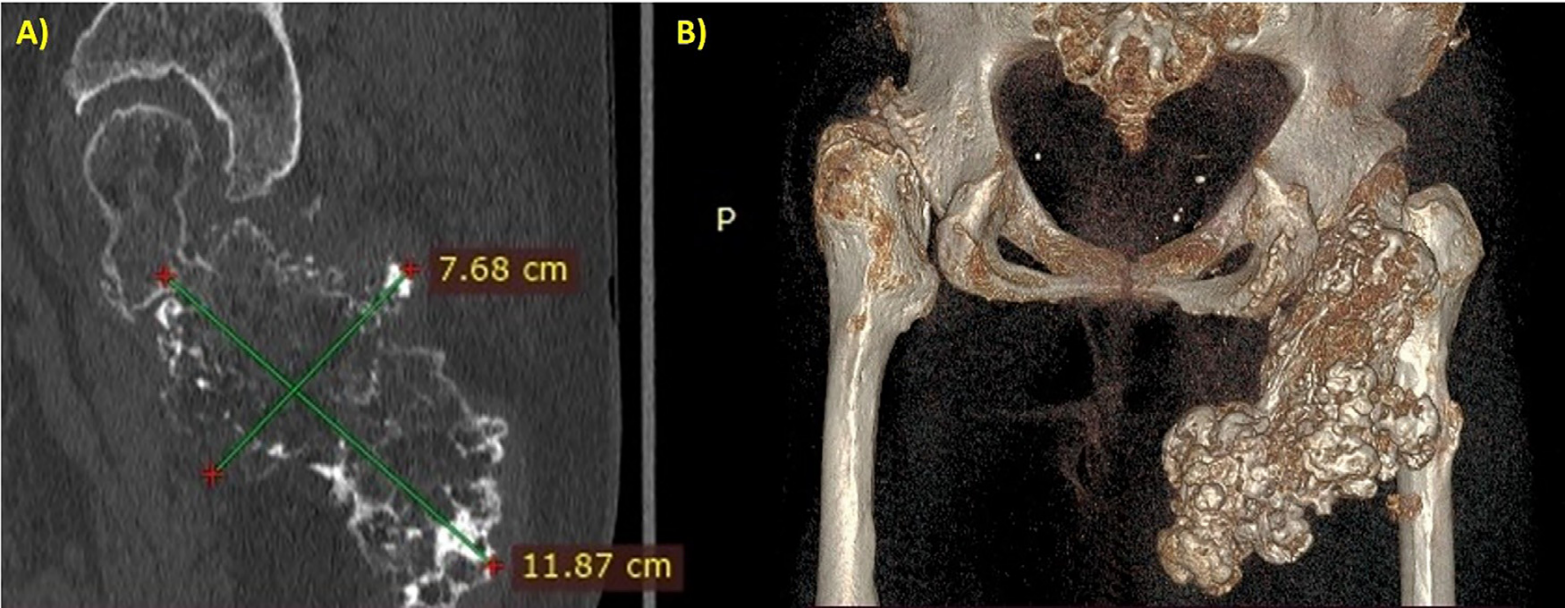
(A, B) The exostosis measured in a sagittal plane of the CT scan (A) and reconstructed in a 3D image (B).
